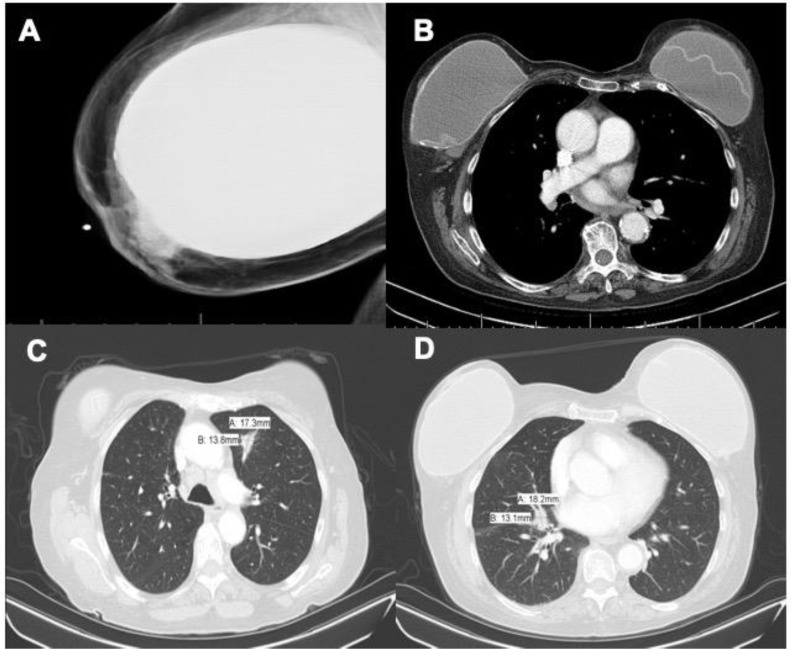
(A) Mammogram demonstrating extracapsular rupture of the right breast, (B) mediastinal window chest CT demonstrating Linguine Sign of the left breast implant, (C) chest CT showing an 18 mm × 14 mm nodule in the right middle lobe causing concern for malignancy, and (D) the same image showing a 17 mm × 14 mm spiculated left lung nodule with concern for breast involvement.
